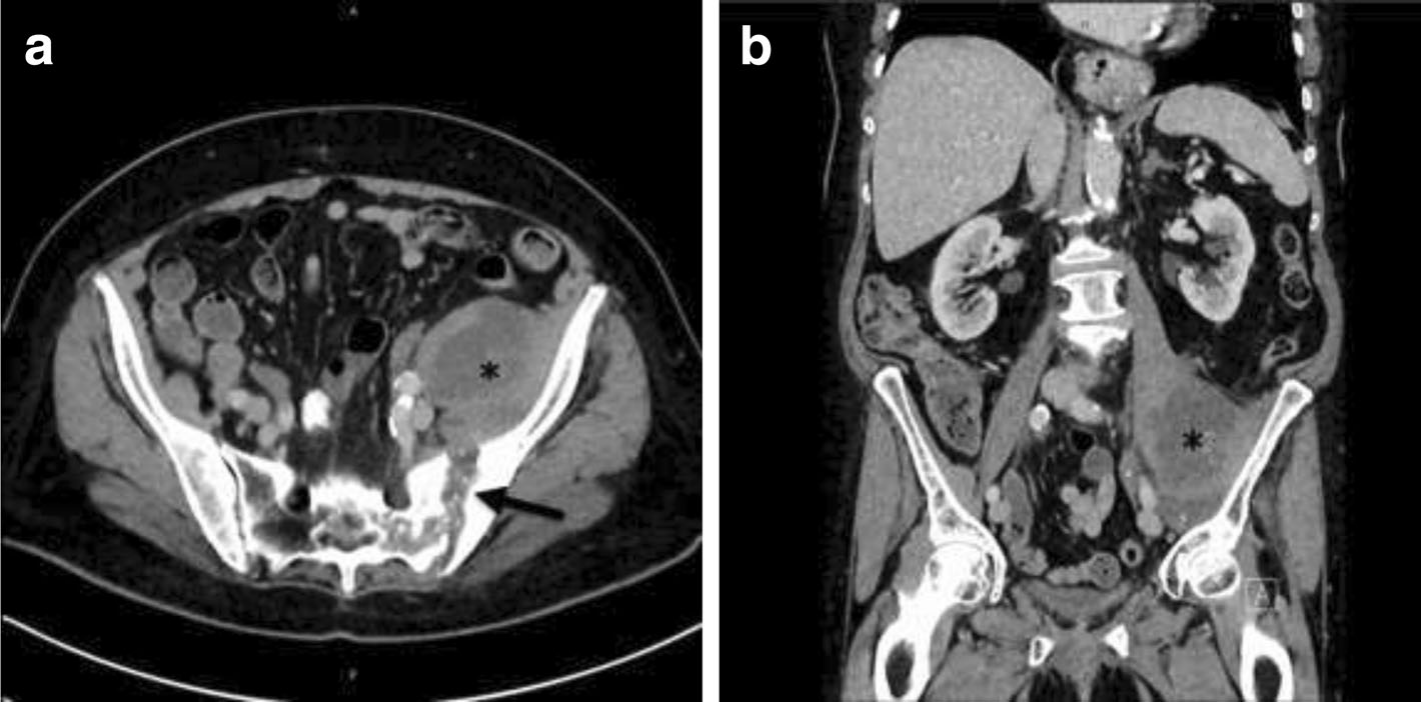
Abdominal computed tomography images showing left psoas abscess (*star*) and destruction of the sacroiliac joint (*arrow*). a Coronal image; b axial image
